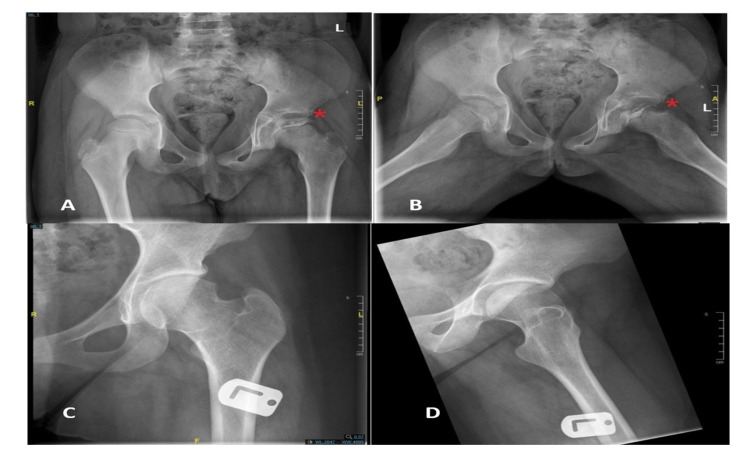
Example of pelvic x-rays before (A, B) and after (C, D) surgical treatment of patient with Legg-Calvé-Perthes disease aged younger than eight years.
